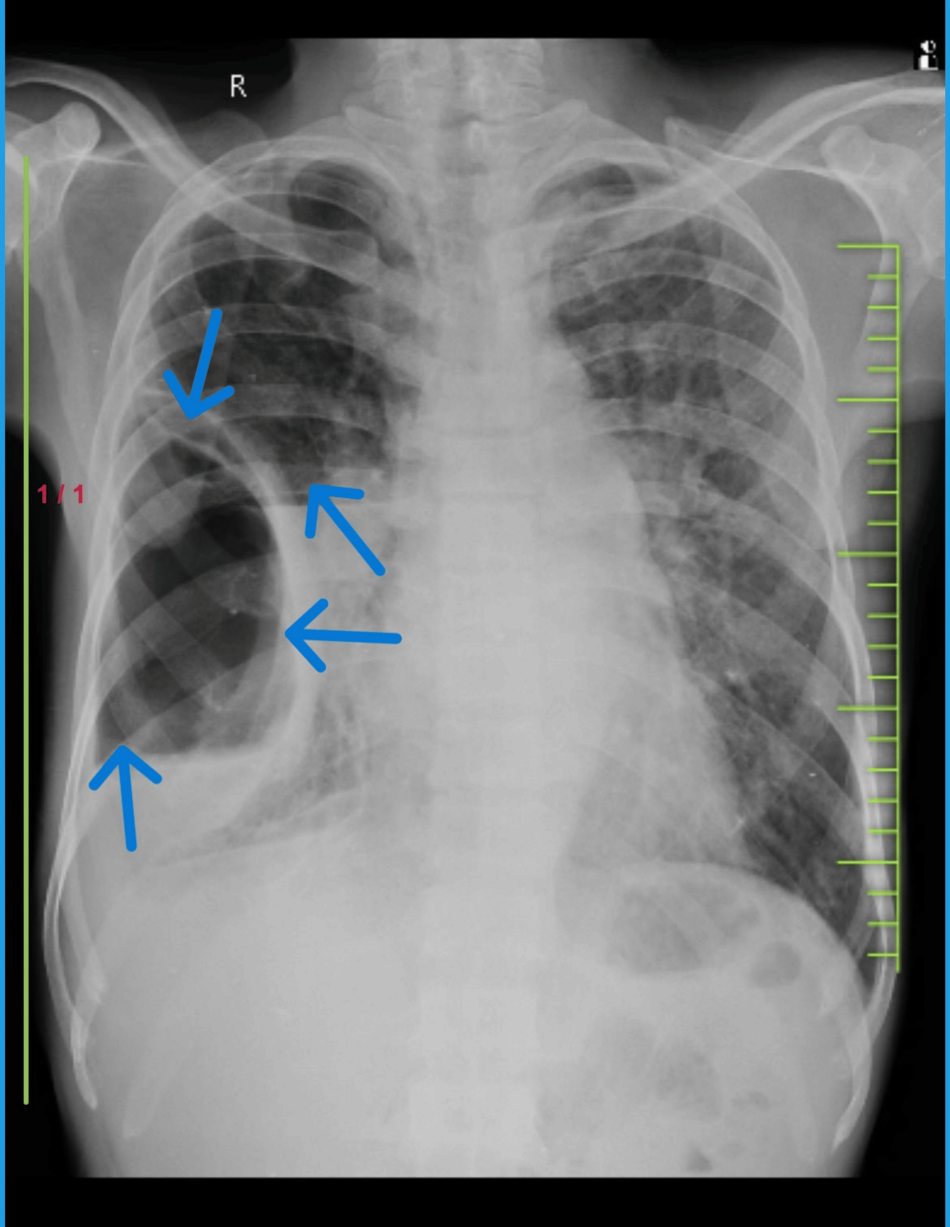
The chest X-ray PA (posteroanterior) view showing a thick-walled bulla with an air-fluid level and underlying atelectasis in the right lung (indicated by an arrow).
